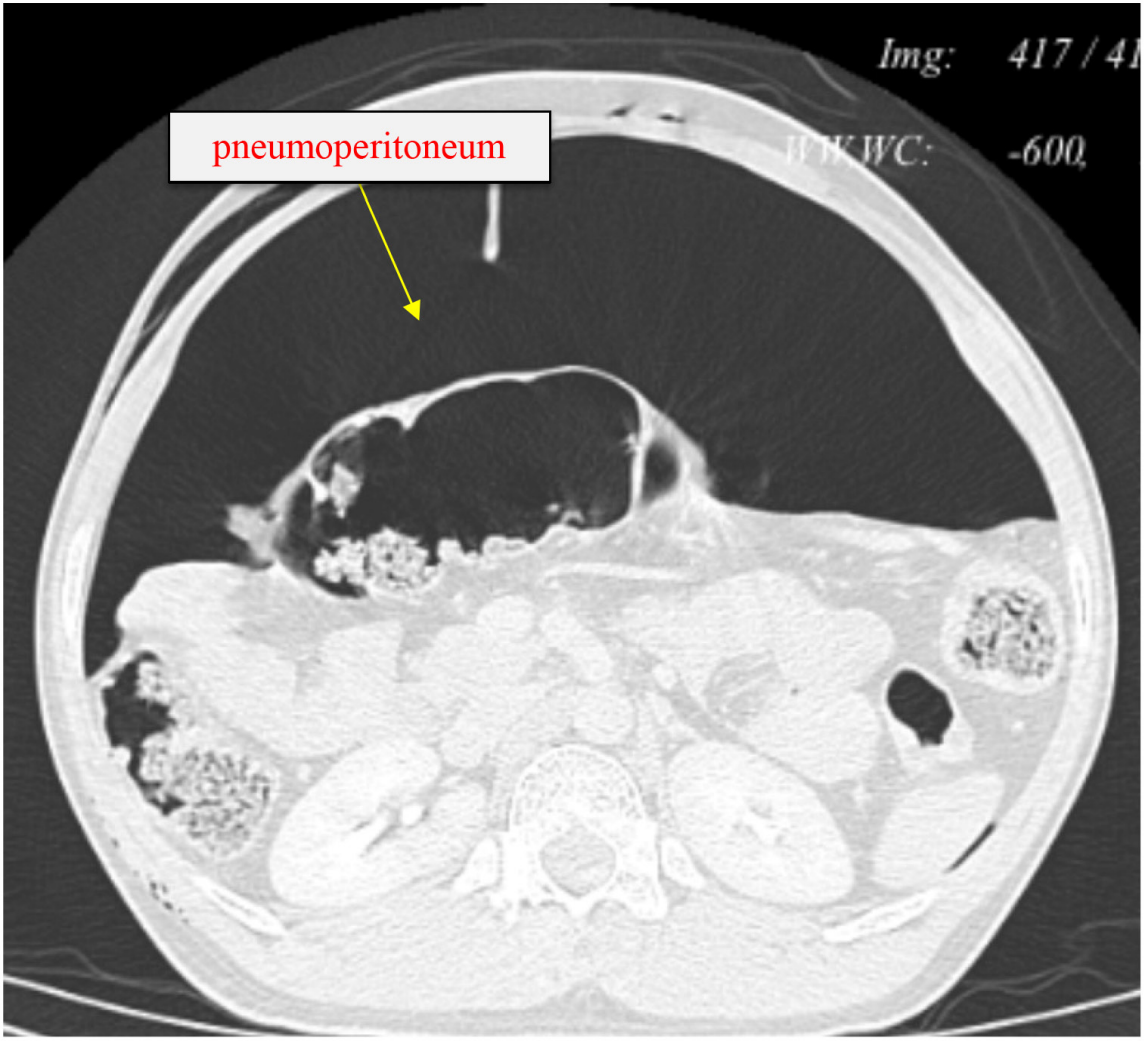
CT abdomen with lung window showing severe pneumoperitoneum predominantly in the anterior aspect causing mass effect over the visceral organs.
